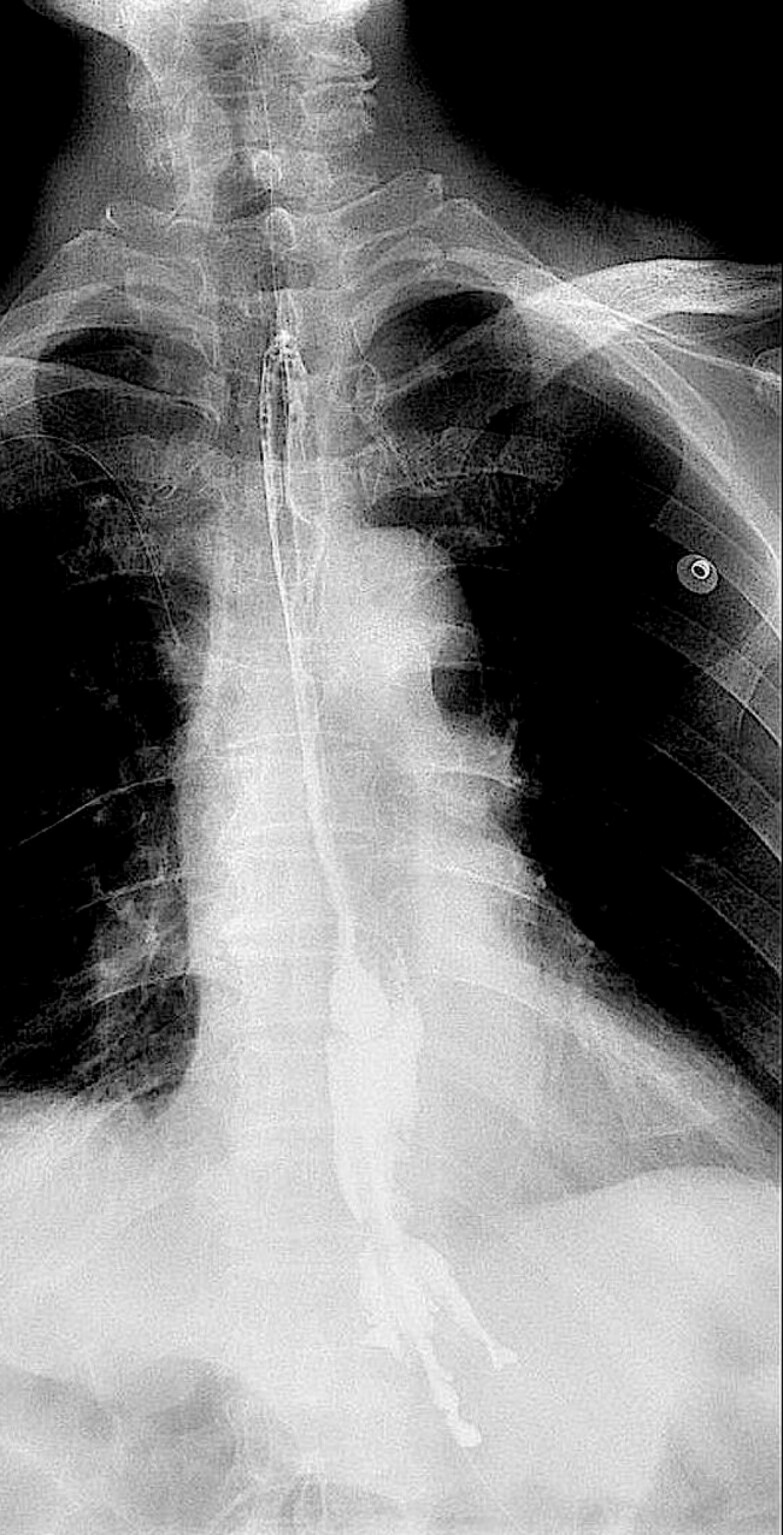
Postoperative upper gastrointestinal radiography.
